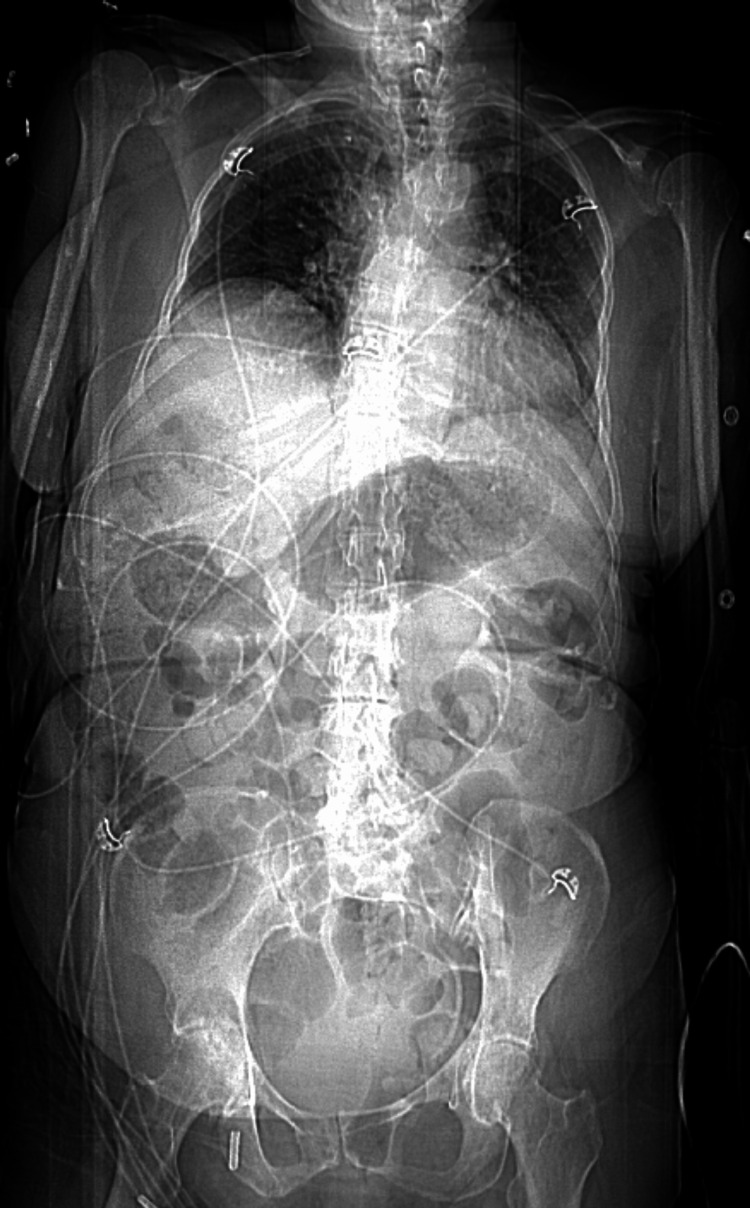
CT of the Thorax/Abdomen/Pelvis (Coronal View) Coronal view of the CT of the thorax/abdomen/pelvis does not reveal overt abnormalities. CT: computed tomography
